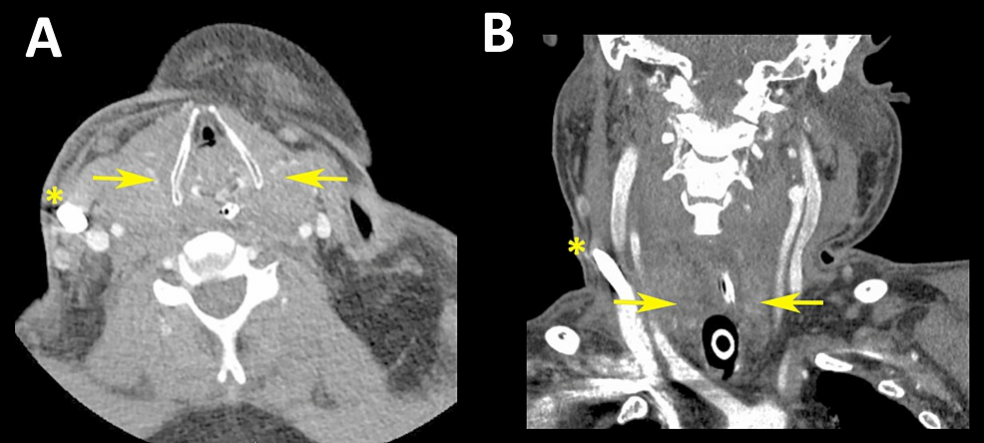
Contrast-enhanced computed tomography images of the neck. Axial view (A) and coronal view (B). The yellow arrows denote the enlargement of the thyroid. The yellow asterisk denotes the position of the extracorporeal membrane oxygenation catheter.
